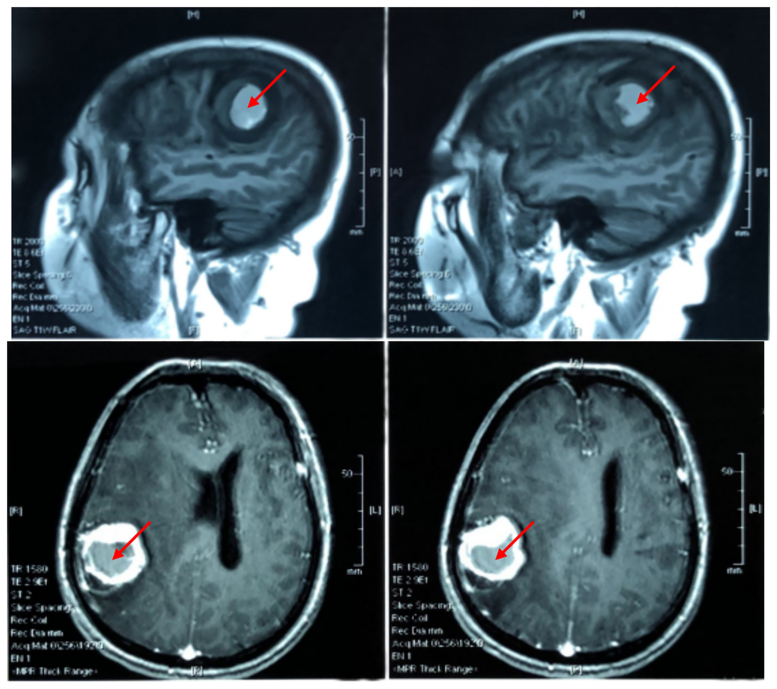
MRI scan of a solid tumoral mass at the right parietal lobe. MRI = magnetic resonance imaging.
